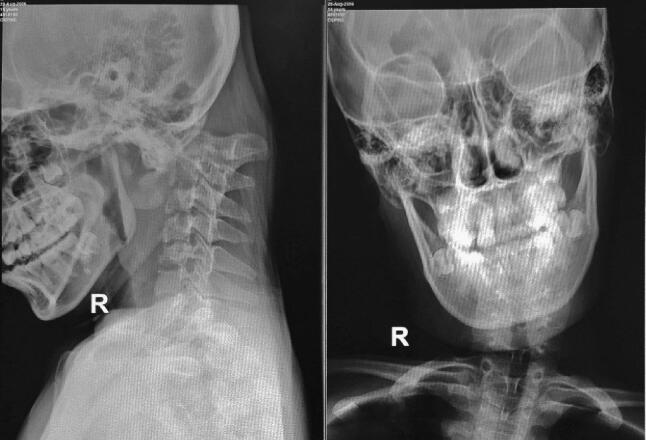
X-ray examination of the cervical spine showed atlantoaxial dislocation with type II odontoid fracture.
